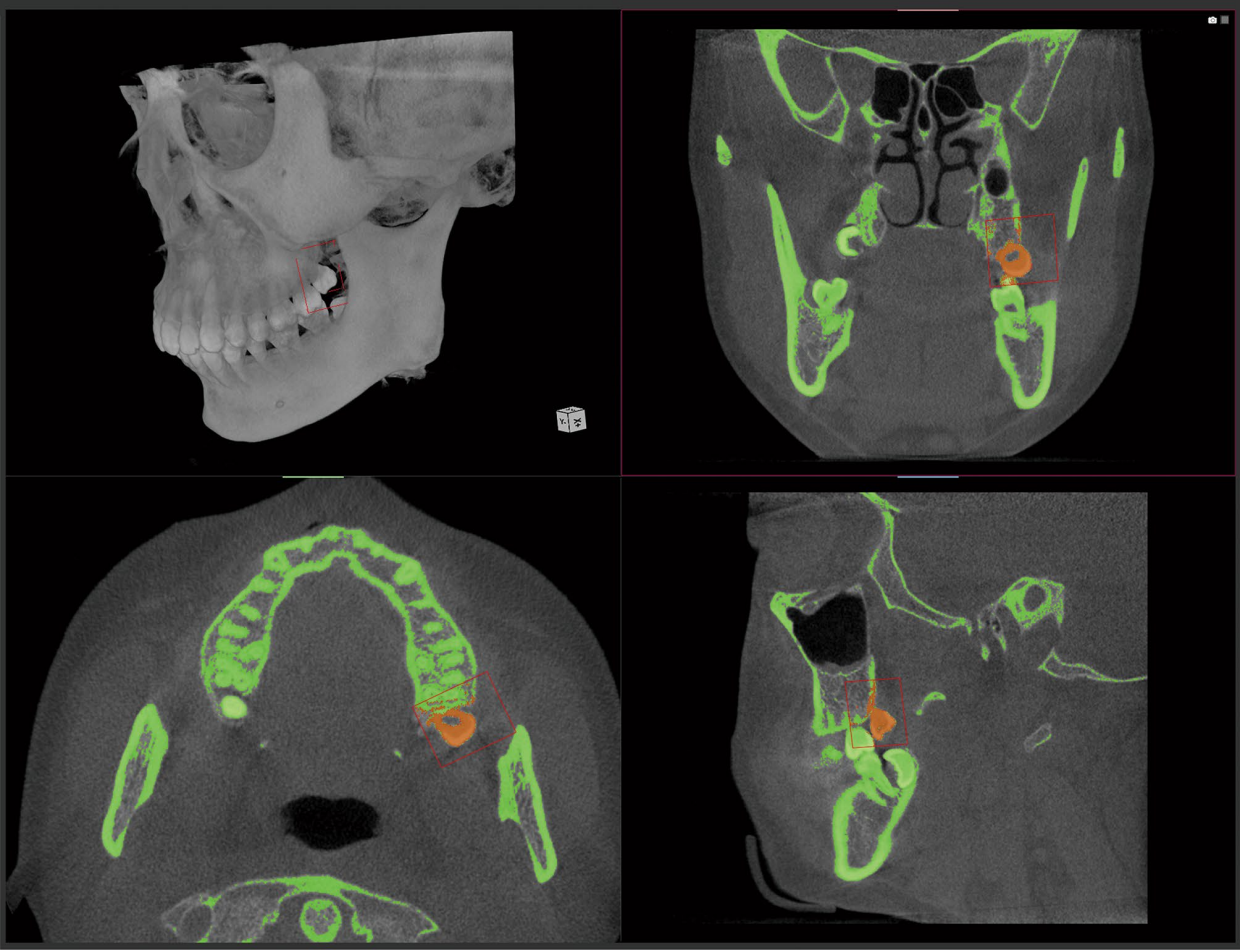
CBCT region segmentation, Boolean subtraction, and HTVC region division. The green region is the result of region segmentation. The orange region is the HTVC region of the maxilla, which the volume is calculated by pixel counting
